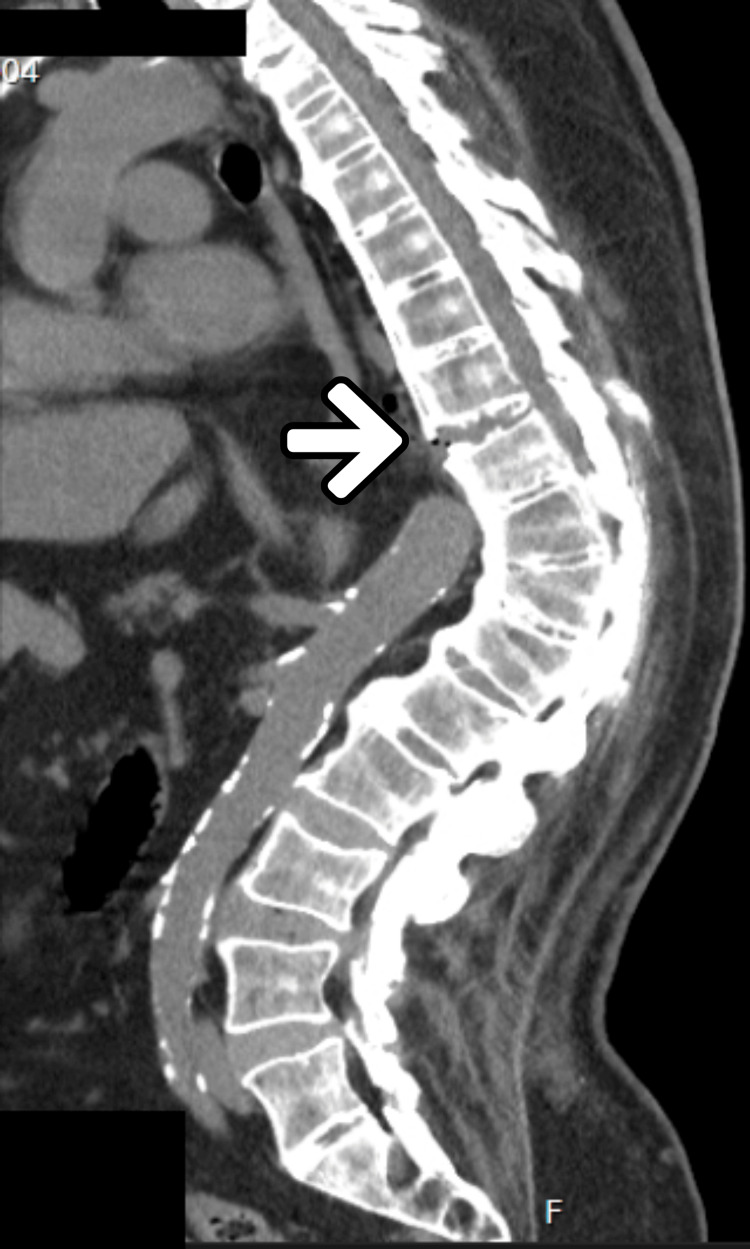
Patient's CT scan revealing important dorsal kyphosis. Arrow: D8-9 vertebral fracture, CT: computerized tomography
